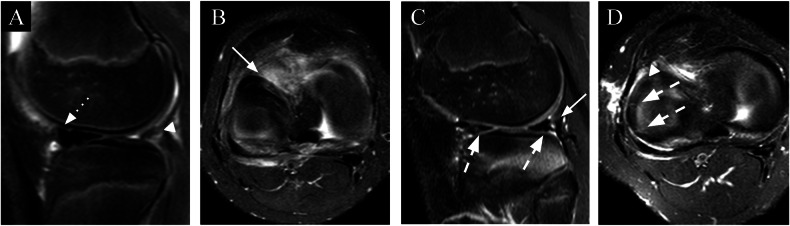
An 11-year-old male with a history of knee trauma and postsurgical suture evaluation 2 months later. A, B Sagittal and axial T2FS: presurgical MR image showing detachment of the posterior horn of the meniscocapsular junction (arrowhead) with anterior displacement (dotted arrow) and thickening of the anterior horn (arrow). C, D Sagittal and axial T2FS: image 2 months postsurgical suturing of the meniscus with reinsertion of the posterior horn showing posterior meniscocapsular repair (arrow) and high signal lines within the body and anterior and posterior horns indicating the suture sites (dashed arrow)
